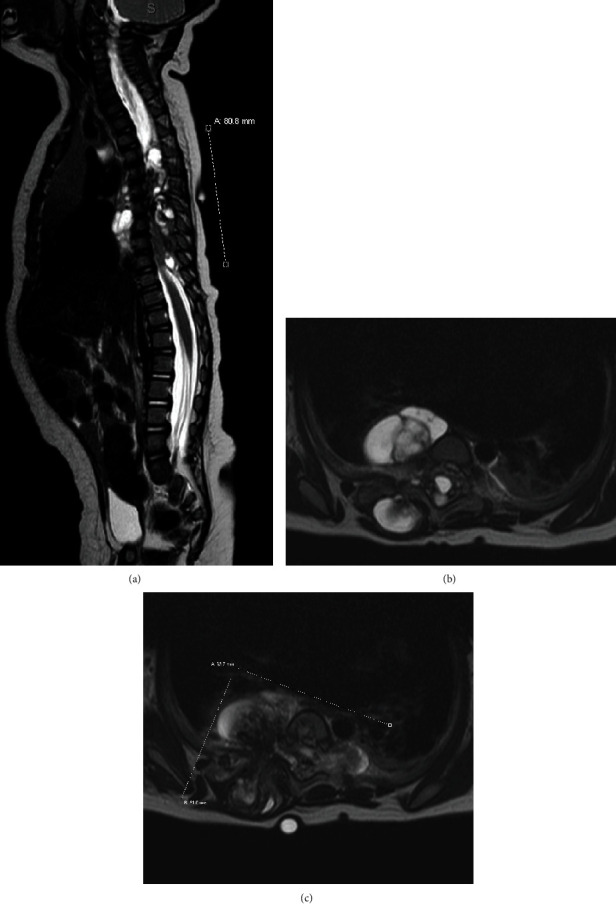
(a) Sagittal T2-weighted MR image showing multilobulated, partially cystic, trans-spatial heterogenous mass extending cranially to T3 and caudally to T10 with soft tissue extension anteriorly into the dorsal aspect of the intrathoracic cavity. (b, c) Axial T2-weight MR image further demonstrating the mass centered at T5-6 paravertebral space extending anteriorly into the dorsal aspect of the intrathoracic cavity, into the right dorsal paraspinal soft tissue/musculature, and into the spinal canal with severe displacement of the cord and resultant spinal stenosis.
